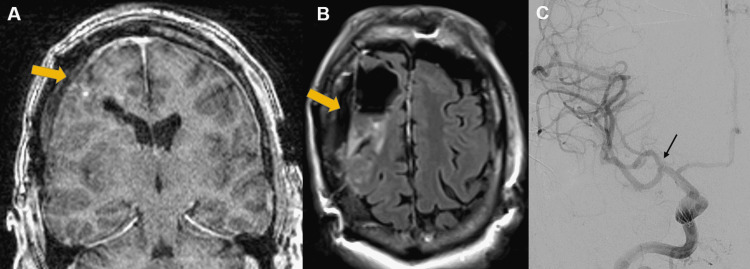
Intraoperative MRI/angiogram images Intraoperative MRI showing gross total resection of the cyst and the cavernous malformation (yellow arrows; A and B), with intraoperative angiogram showing complete exclusion of the aneurysm from the circulation without parent vessel compromise (black arrow; C).
